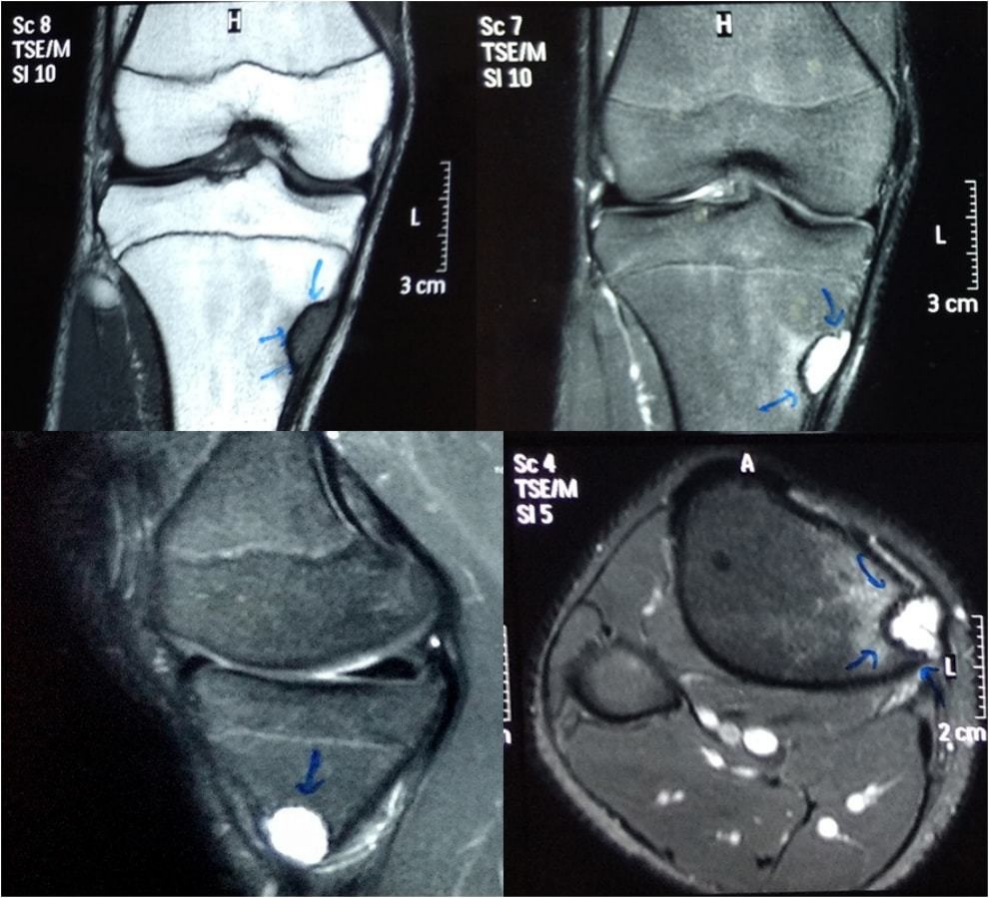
MRI images of the site of the lesion The images show hypointense on T1 and hyperintense on T2.
